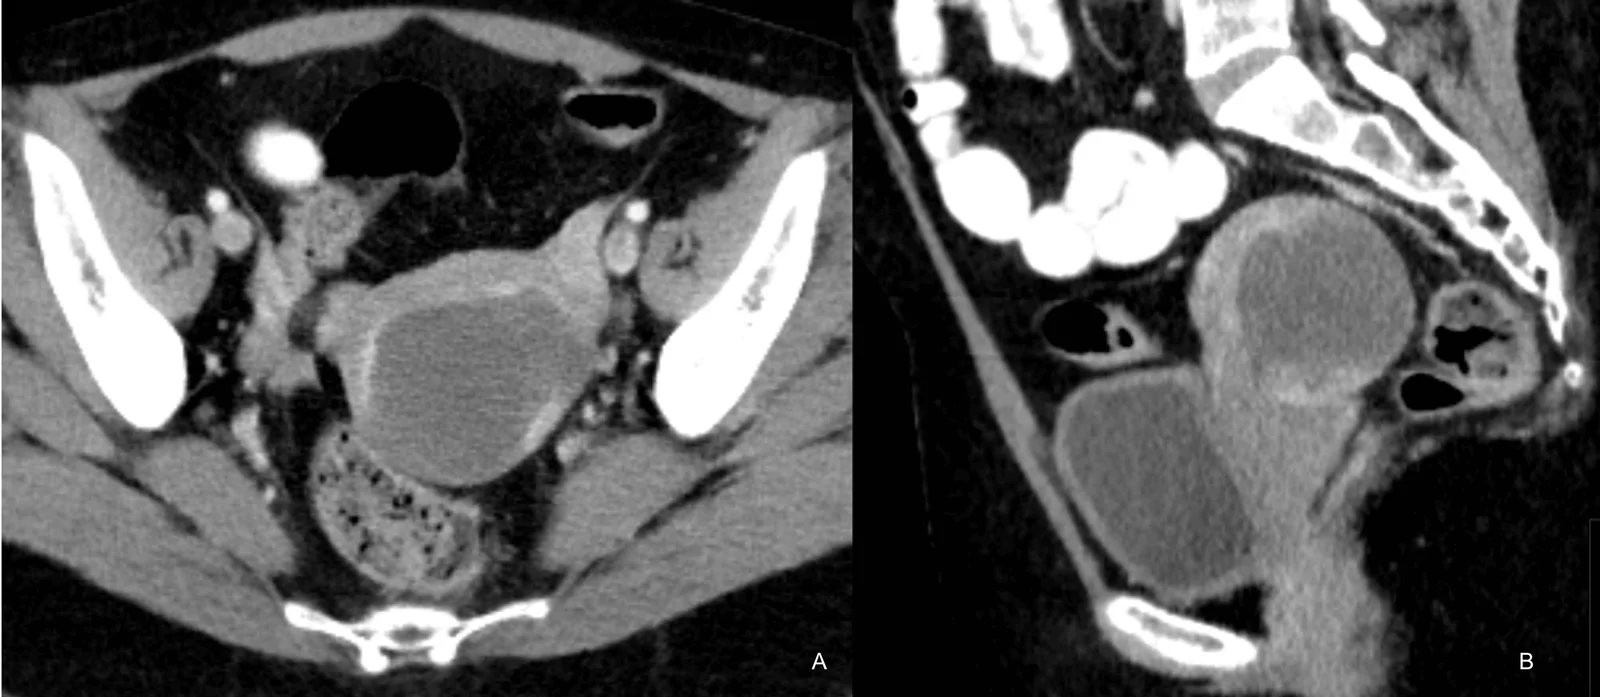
CT of the pelvis demonstrating persistence of the uterine tumour following chemotherapy. Axial (A) and sagittal reformatted (B) CT images of the pelvis both demonstrate persistence of the uterine tumour following chemotherapy.
